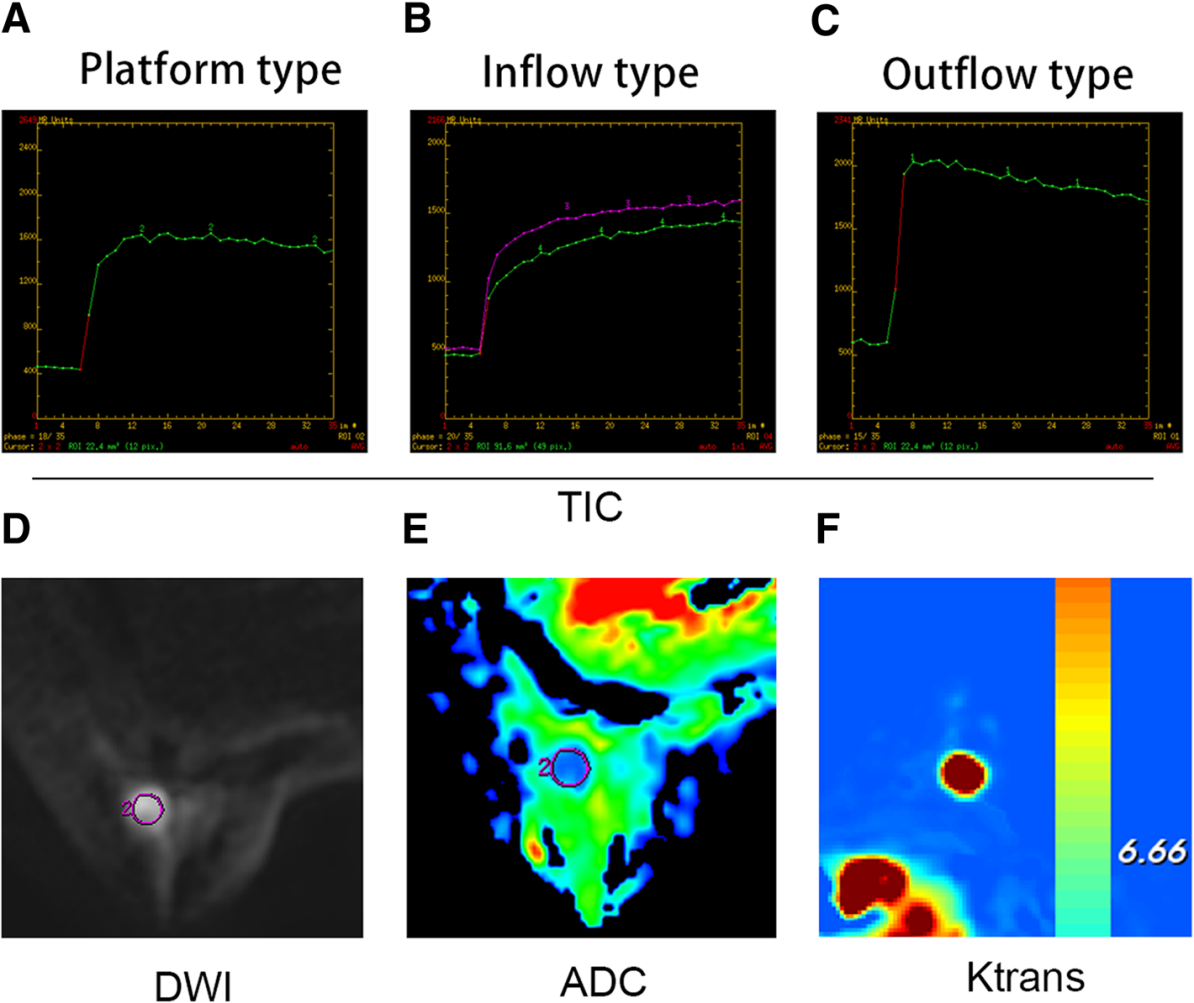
a Platform type TIC curve. b Inflow type TIC curve. c Outflow type TIC curve. d Diffusion-weighted imaging (DWI) map. e Apparent diffusion coefficient (ADC) image. f Ktrans image
